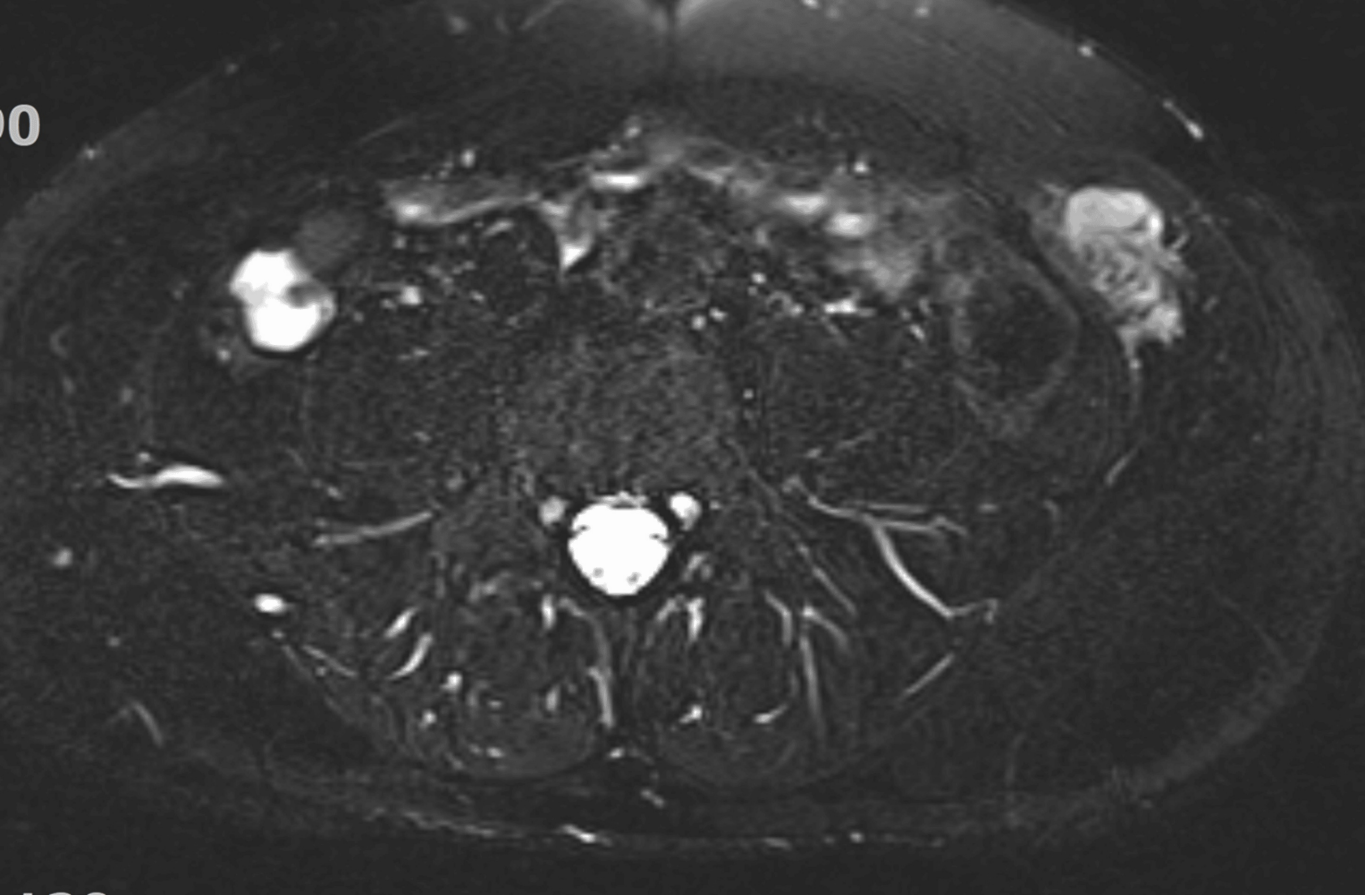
MRI axial T2 FAT SAT (fat-saturation) Heterogeneous hyperintensity T2 space occupying mass in the left internal oblique muscle.
